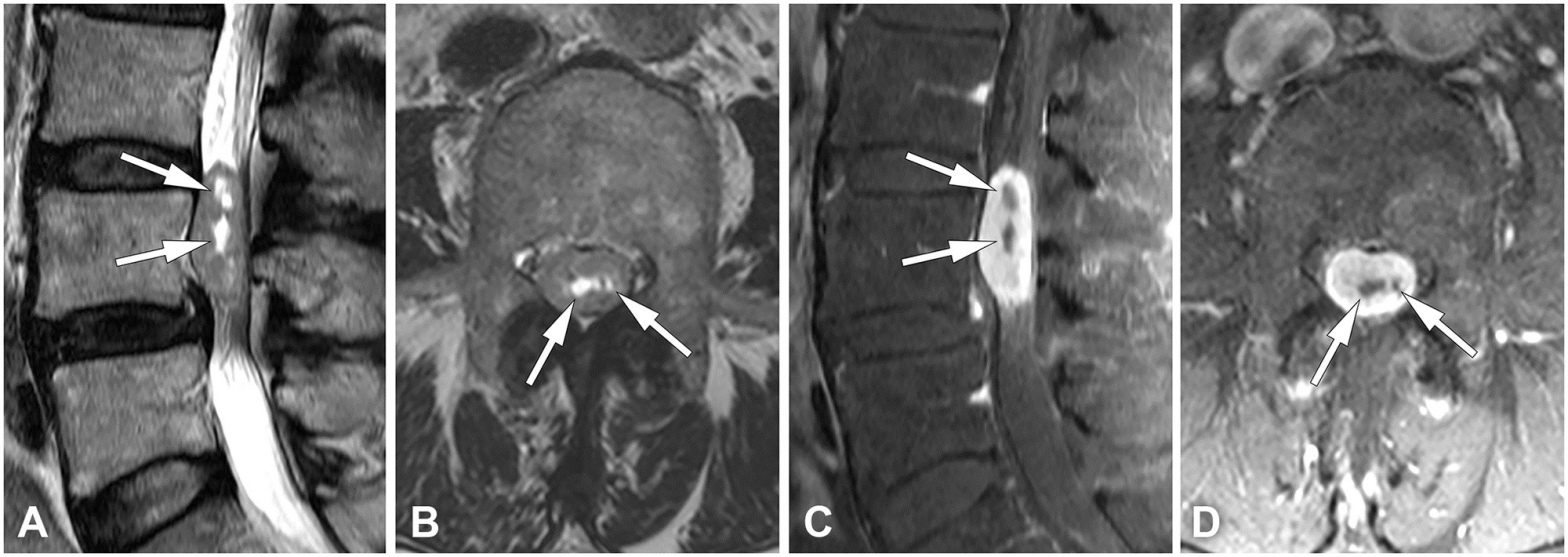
A schwannoma in a 59-year-old woman. (A) Sagittal and (B) axial T2-weighted images show an intradural extramedullary mass on the L4 level with measurable cyst formation (arrows). (C) Sagittal and (D) axial fat-suppressed contrast-enhanced T1-weighted images show relatively homogeneous enhancement except for non-enhancing cystic portions (arrows).
